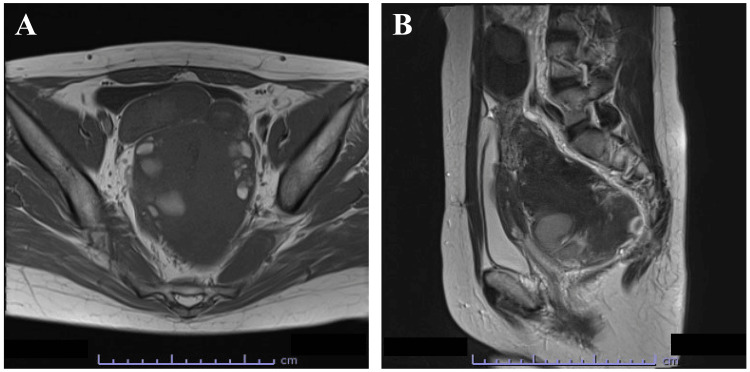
T1-weighted magnetic resonance imaging findings in the pelvic tumor. (A) Horizontal view. (B) Sagittal view. The size of the largest leiomyoma nodule in the pouch of Douglas was 14.5 x 9.5 x 9.4 cm. The hemorrhagic cysts inside the tumor were located in the pouch of Douglas, and the continuity with the uterus was unclear. Both ovaries and fallopian tubes were normal. Ascites was not observed.
